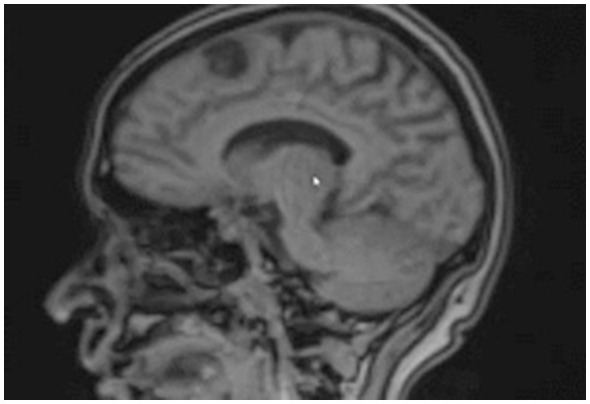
Non-contrast brain MRI – axial and coronal T2-weighted sequence.
